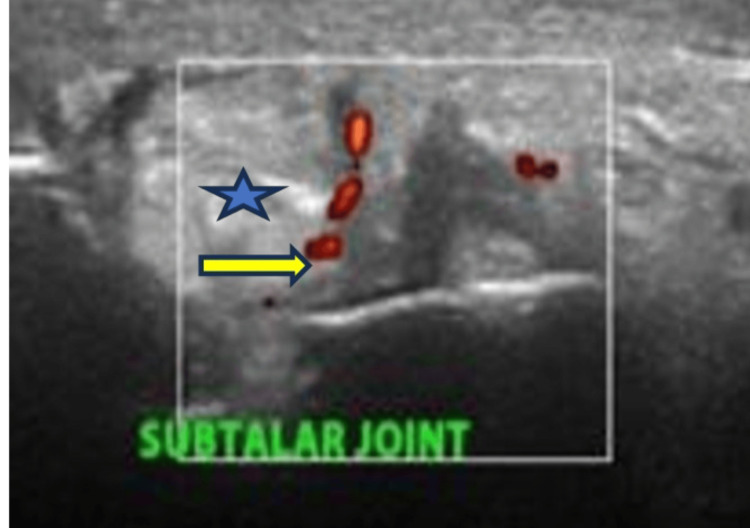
Ultrasound findings of the subtalar joint on the medial transverse view showing synovial hypertrophy with a grade 2 Doppler signal The yellow arrow indicates a grade 2 Doppler signal; the green star indicates synovial hypertrophy.
